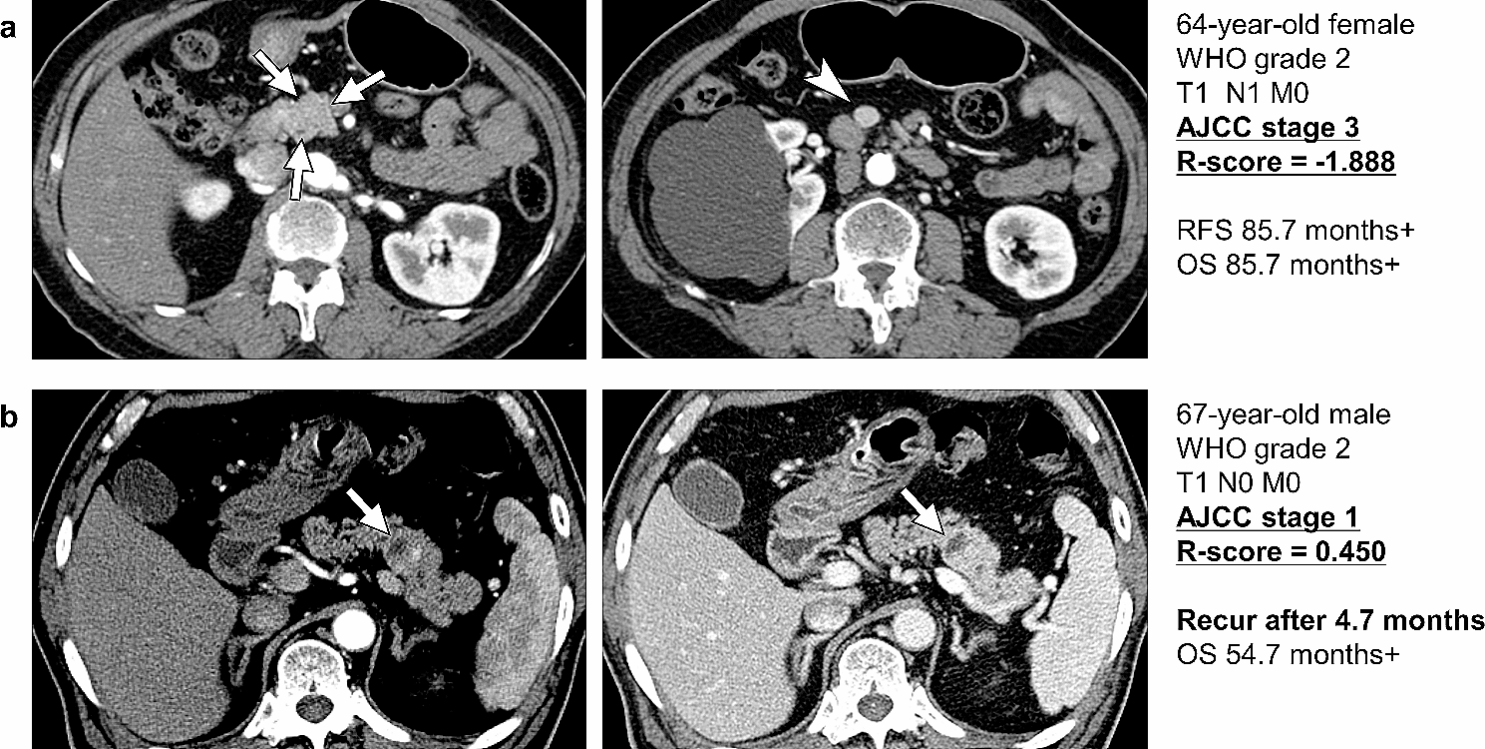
Representative cases of PanNET patients. (a) Preoperative AP CT images of a 64-year-old woman show a 1.8-cm homogeneously enhancing mass at the head of the pancreas (arrows). Regional lymph node metastasis is noted (arrowhead). This tumor was a WHO grade 2 PanNET with AJCC stage III. Although the initial tumor stage was high, the patient survived without recurrence until the last follow-up date (85.7 months after surgery). The R-score of this tumor was − 1.888. (b) Preoperative AP and PVP CT images of a 67-year-old man show a 1.9-cm mass at the tail of the pancreas. The mass shows heterogeneous enhancement, especially on AP images (arrows). This tumor was a WHO grade 2 PanNET with AJCC stage I. Despite the low AJCC stage, recurrence occurred at 4.7 months after surgery. The R-score of this tumor was 0.450, obviously higher than that of the patient in (a). AJCC, American Joint Committee on Cancer staging system; AP, arterial phase; OS, overall survival; PanNET, pancreatic neuroendocrine tumor; PVP, portal venous phase; RFS, recurrence-free survival; R-score, radiomics score; WHO, World Health Organization
